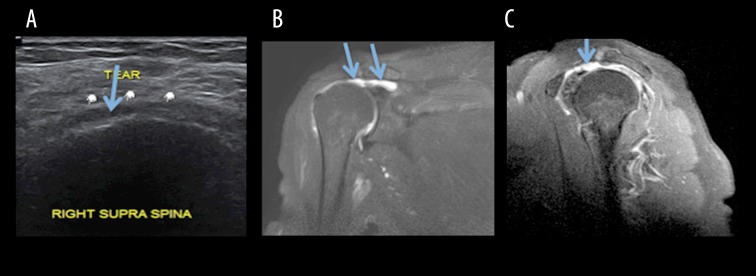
(A) USG image showing a complete tear of the supraspinatus tendon (B) MRI, T2W coronal image (C) T2W, sagittal image depicting a full-thickness tear of the supraspinatus tendon (arrow marked).
